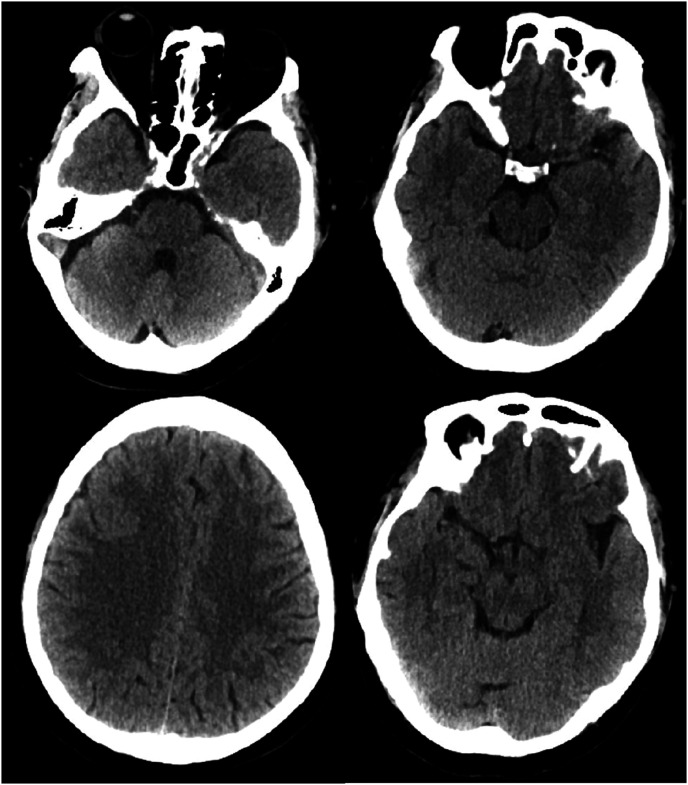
Emergency cranial computed tomography scan showing no evidence of bleeding.
